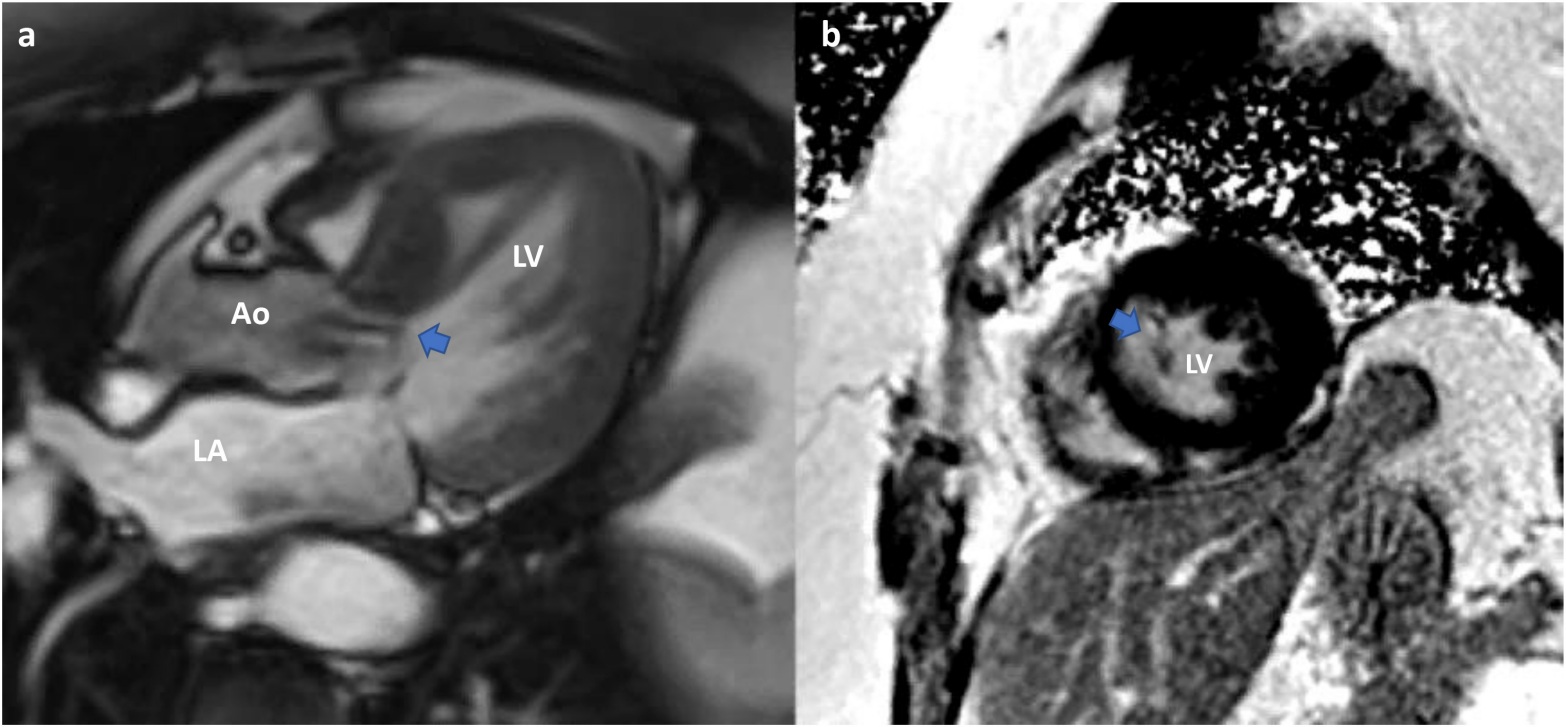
Cardiac MRI. Planning of alcohol septal ablation in patient with hypertrophic cardiomyopathy and severe mitral regurgitation due to systolic movement of the anterior mitral valve leaflet (SAM). (a) SSFP-cine Three chamber: Systolic motion of the anterior MV with intracavitary flow acceleration (diphasic) and consequent significant mitral regurgitation. Asymmetrical septal hypertrophy. (b) T1-weighted late gadolinium enhancement (LGE-MRI) Short axis mid ventricular showing late gadolinium hyperenhancement of the papillary muscle-chordal apparatus (arrow) due to fibrosis secondary to traumatic contact with the septum.
